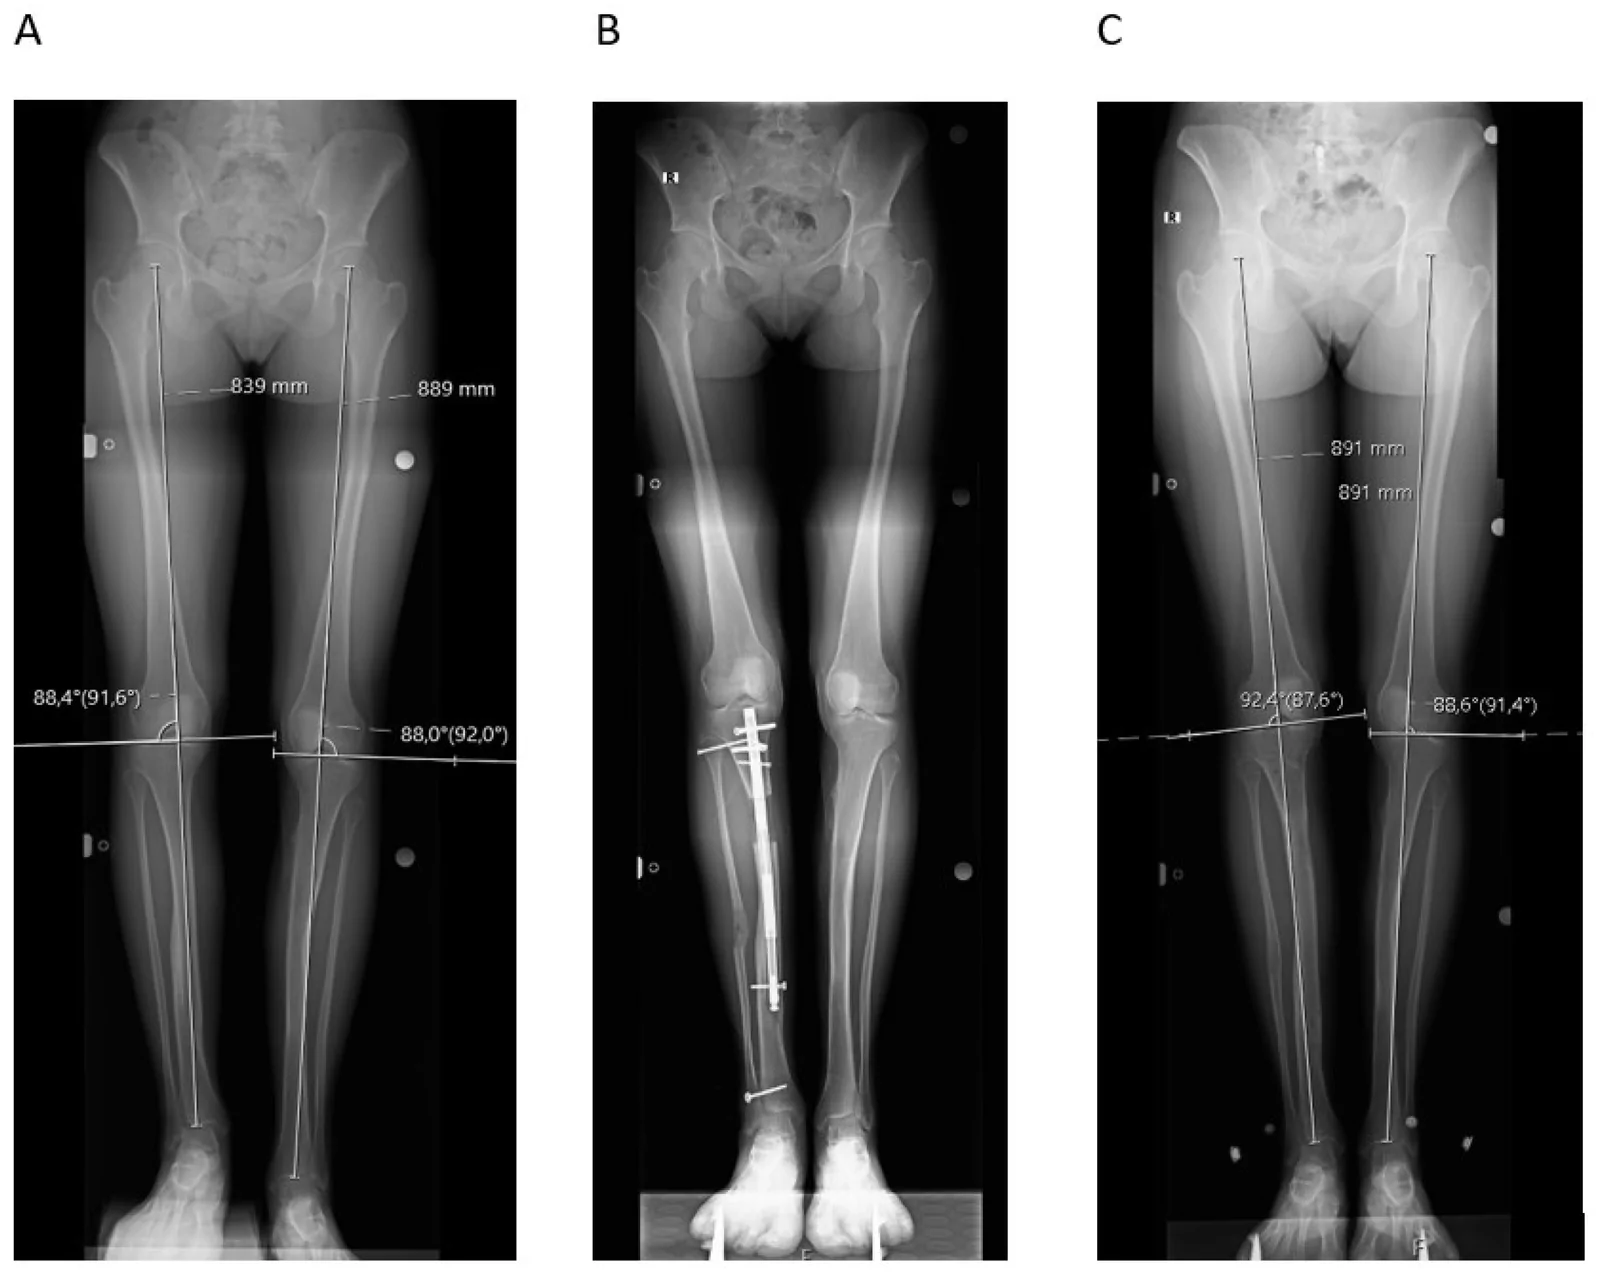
Representative case of tibial lengthening and deformity correction using a magnetically controlled intramedullary nail in a 17-year-old patient with lower extremity length discrepancy. (A) Preoperative standing long-leg radiograph demonstrating tibial shortening, limb length discrepancy, and coronal plane deformity. Preoperative alignment parameters included an mLDFA of 92.4° and an mMPTA of 88.6°. (B) Radiograph obtained during the distraction/consolidation phase following tibial osteotomy and insertion of a magnetically controlled intramedullary lengthening nail. (C) Final standing long-leg radiograph after completion of treatment demonstrating successful limb length equalization, satisfactory regenerate consolidation, restoration of lower extremity alignment, and improvement of the coronal plane deformity. Final alignment parameters were mLDFA 88.4° and mMPTA 88.0°.
